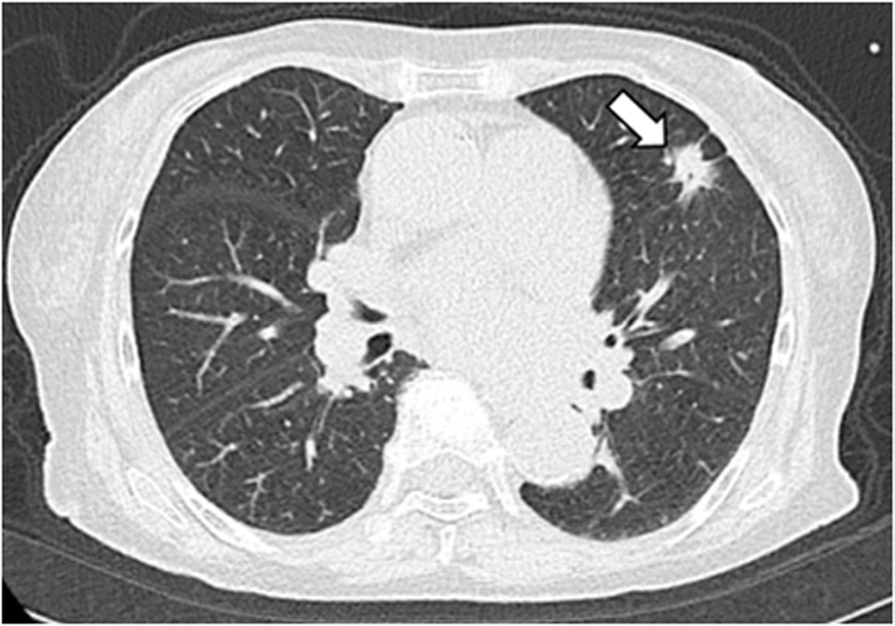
Preoperative computed tomography shows a nodular shadow of 1.4 cm (arrow) under the pleura of the S3 area of the left lung
